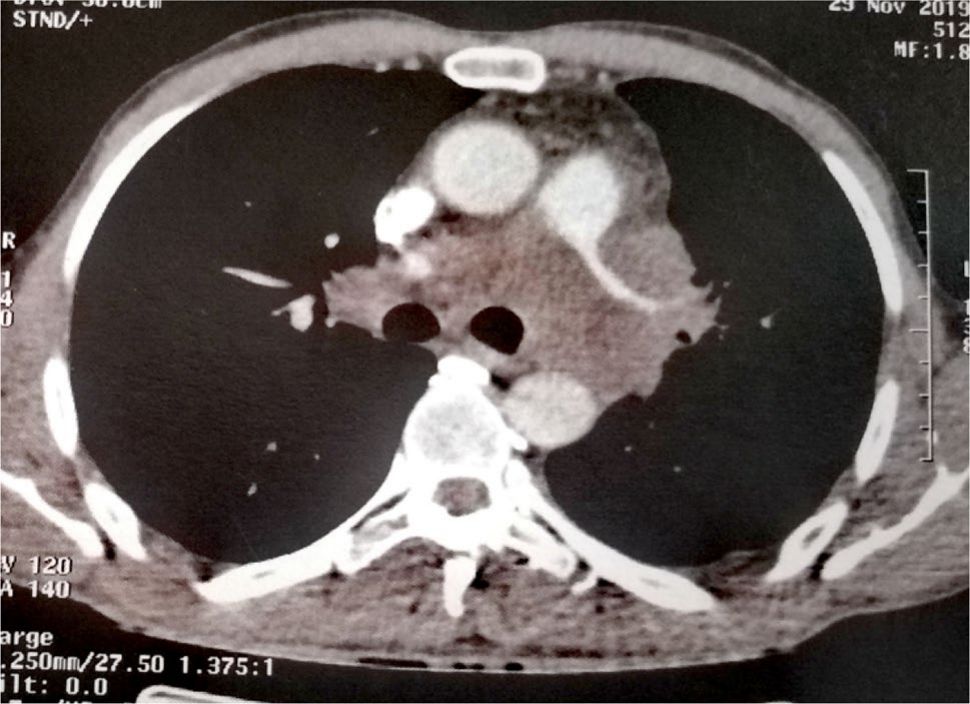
Computed tomography scan of SMARCA4-deficient thoracic sarcomas showed large mediastino–pulmonary mass encompassing left arteries, left common carotid and aortic arch. The mass repressed superior vena cava. This mass was inseparable from latero-tracheal adenomegalies
